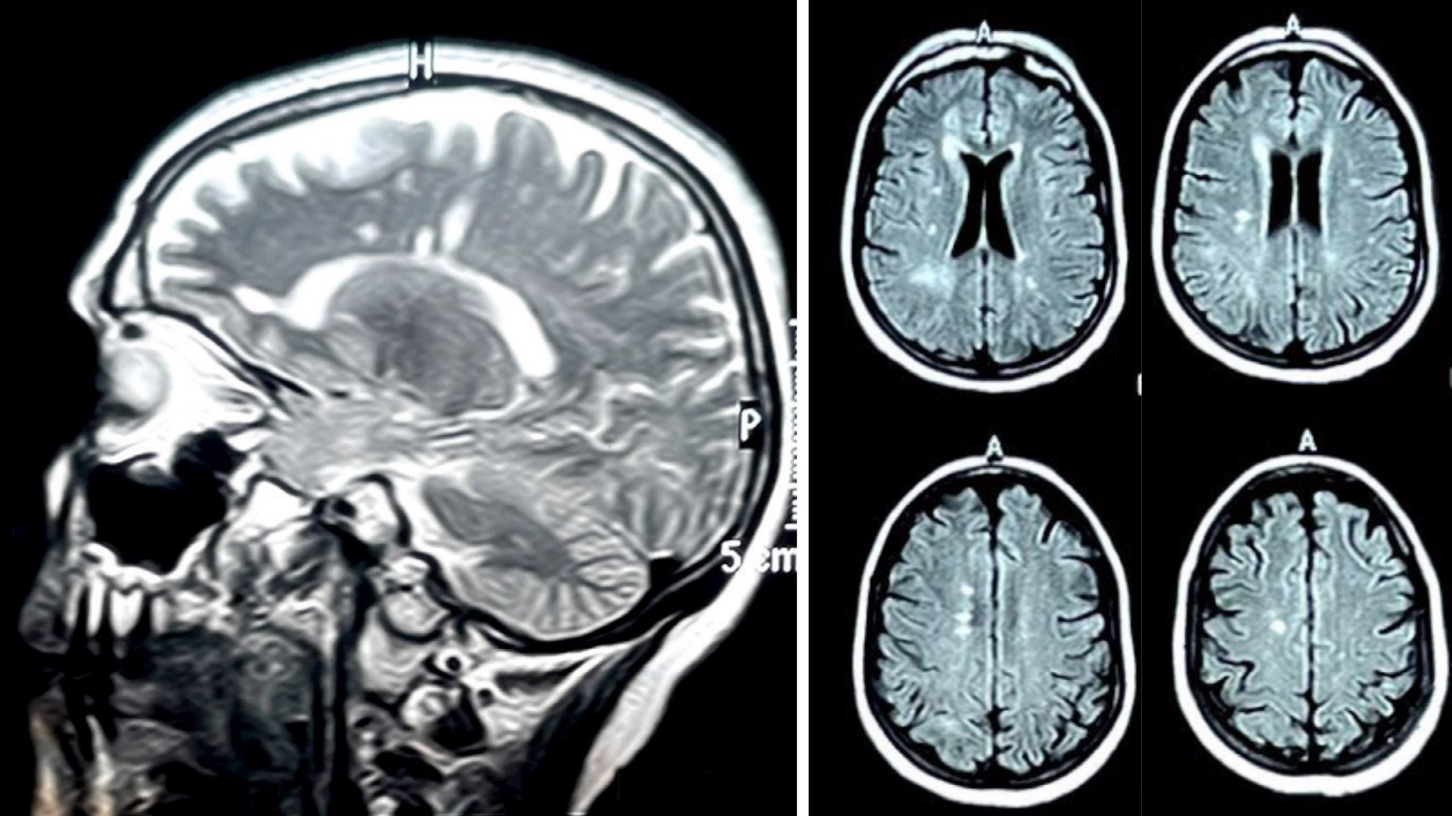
Case 2: sagittal T2‐weighted and axial FLAIR brain MRI sequences showing typical MS lesions in periventricular (Dawson fingers) and juxtacortical regions.
